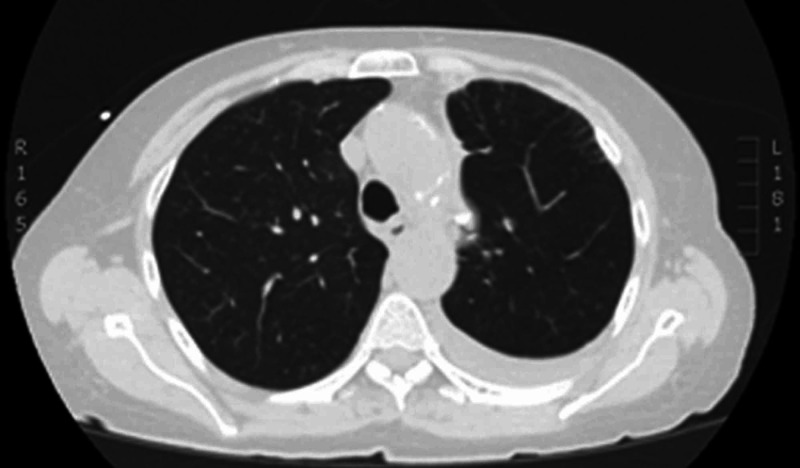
Non-contrast CT scan of the chest status-post talc pleurodesis demonstrating resolution of the secondary spontaneous pneumothorax.
